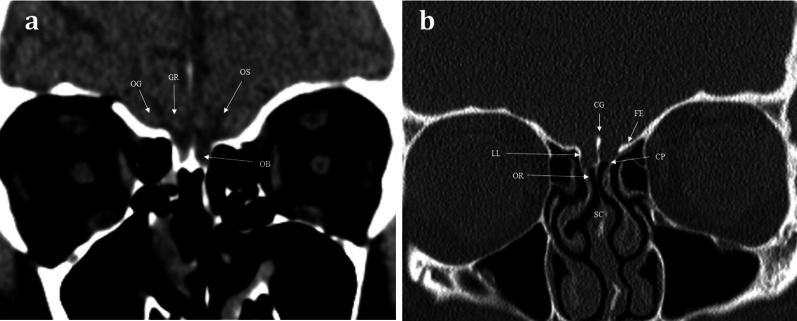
Normal anatomy. Coronal soft tissue (a) and bone window (b) CT images at the level of the olfactory bulb, demonstrating the normal anatomy of the sinonasal compartment (SC) and anterior skull base. The olfactory nerves within the SC and olfactory recess (OR) ascend intracranially via perforations within the cribriform plate (CP). The CP is bounded laterally via the lateral lamella (LL), the crista galli (CG) is seen as a midline projection, and the fovea ethmoidalis (FE) forms the roof of the ethmoid. The position of the olfactory bulb (OB) within the olfactory groove is just discernible on the soft tissue window image (a). The gyrus rectus (GR) and orbital gyrus (OG) are separated by the olfactory sulcus (OS)
